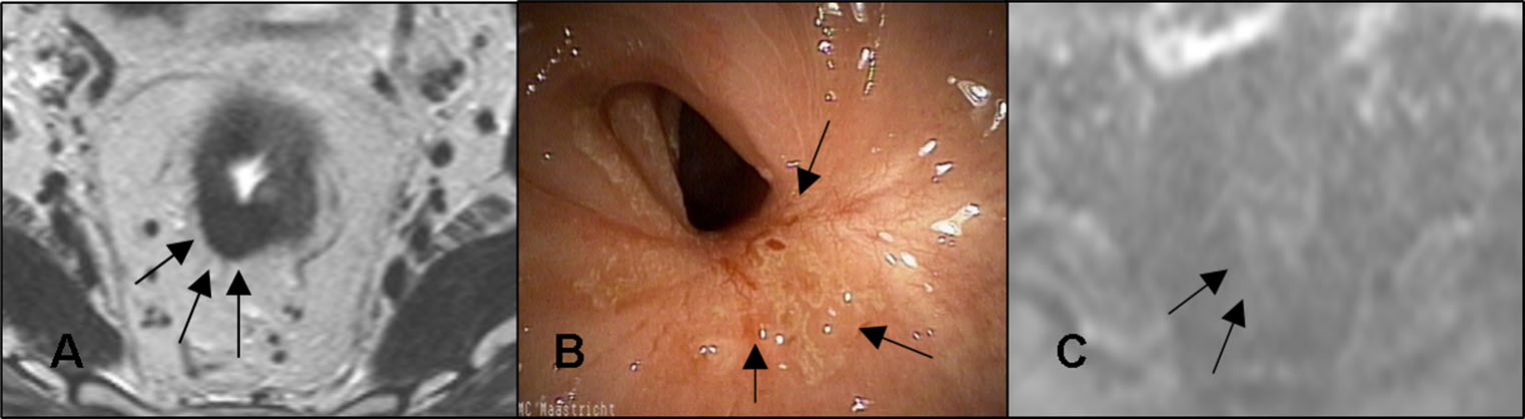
Example of patient with a CR where T2W-MRI (a) revealed marked hypointense residual wall thickening resulting with an equivocal (confidence level 2) score. Clinical assessment (b) revealed a white scar with some stenosis and distortion, and small superficial ulceration, also resulting in an equivocal score. DWI (c) revealed absence of diffusion restriction indicating CR
